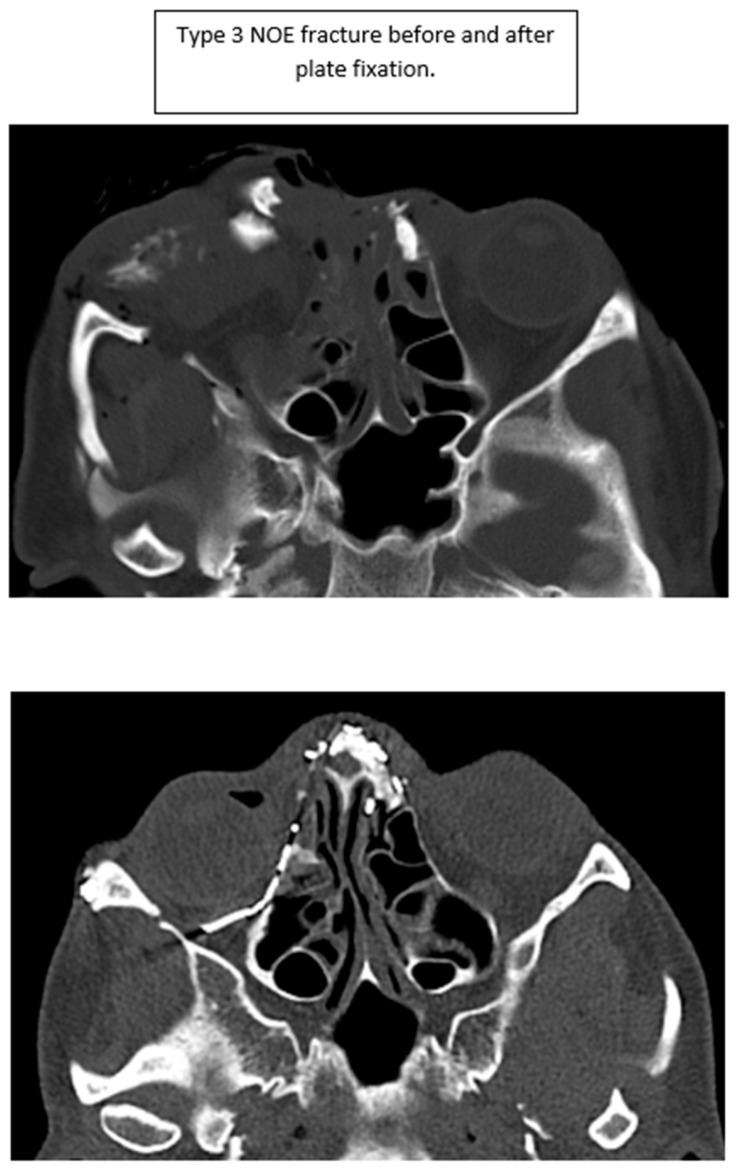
Type 3 NOE fracture CT imaging before and after plate fixation.
